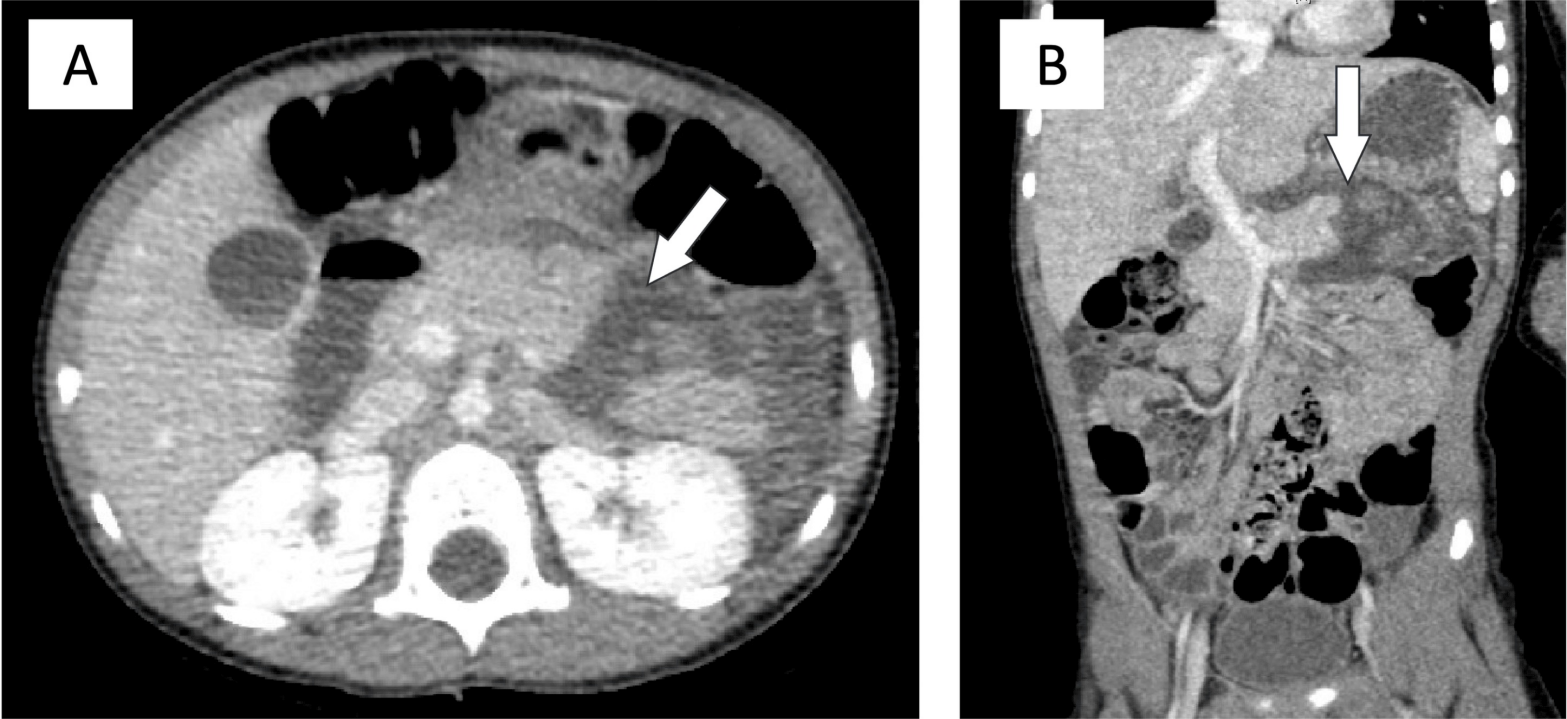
Case 2: Abdominal CT with contrast. (A) Axial view. A ductal injury and complete transection at the junction of the pancreatic body and tail (white arrow), with a thinly encapsulated fluid collection surrounding the transection. Findings were consistent with a high suspicion of a grade III pancreatic injury. (B) Coronal view.
